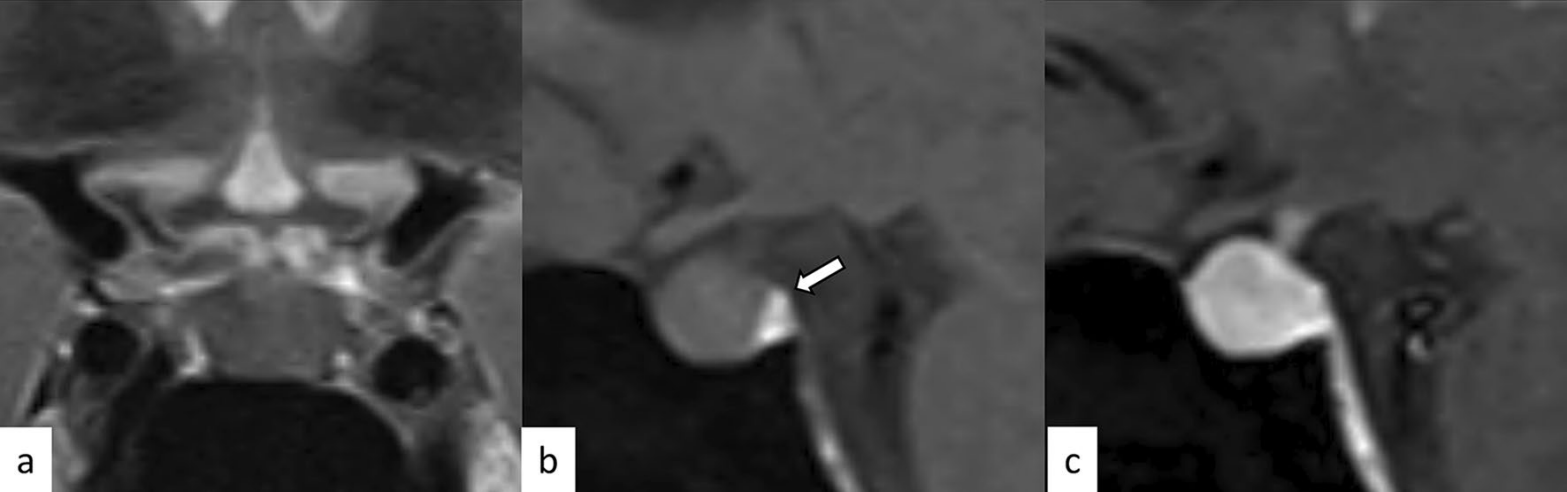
A 64-year-old woman status post-oophorectomy with pituitary hyperplasia. The pituitary gland is symmetrically enlarged with normal signal intensity on T2- and T1-weighted images a, b and homogeneous contrast enhancement (c). The posterior pituitary T1-weighted bright spot is preserved (b, arrow)
